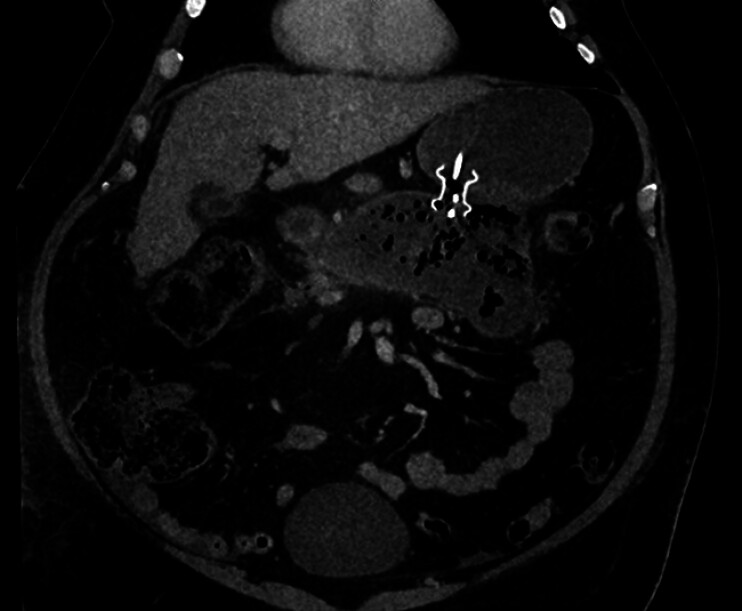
Computed tomography scan showing the lumen-apposing metal stent located across the cystgastrostomy and large walled-off necrotic collection.
